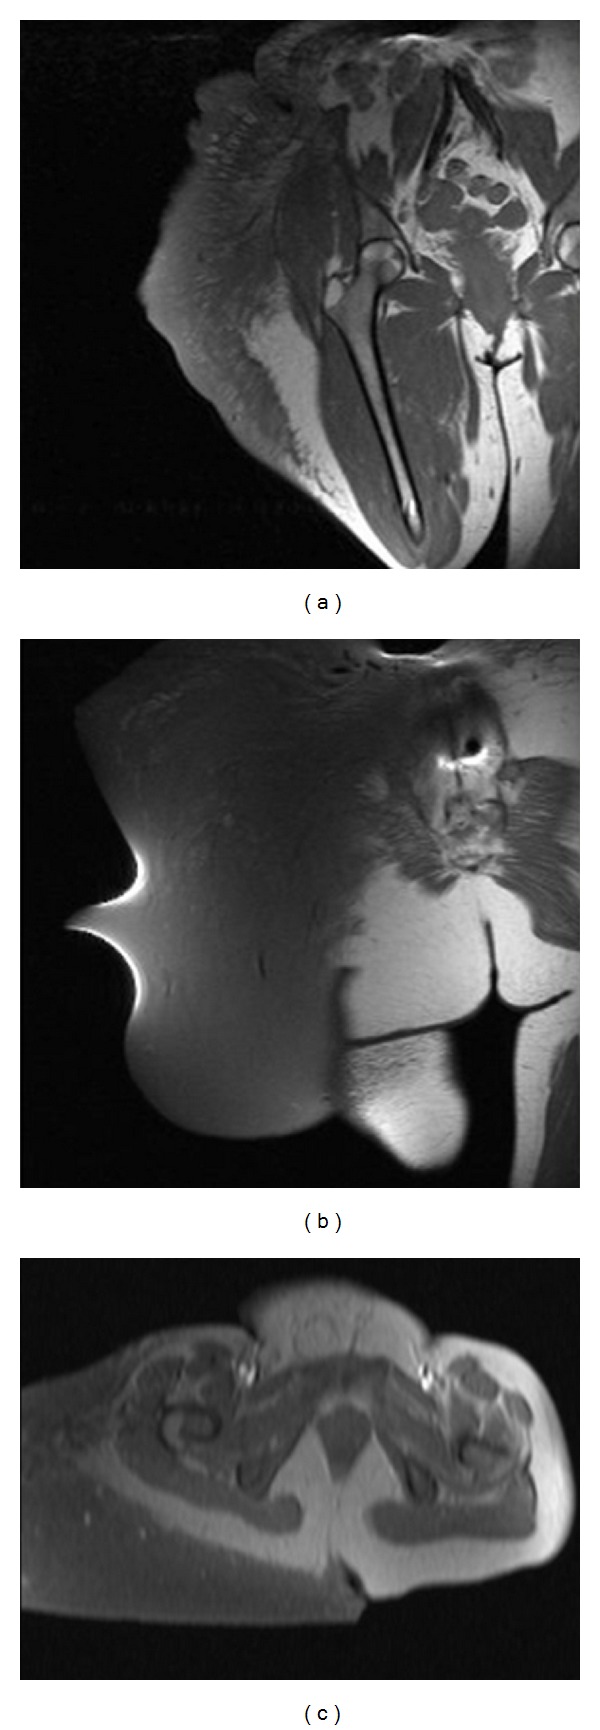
Preoperative MRI images. MRI was technically difficult due to the dimensions of the tumor.
